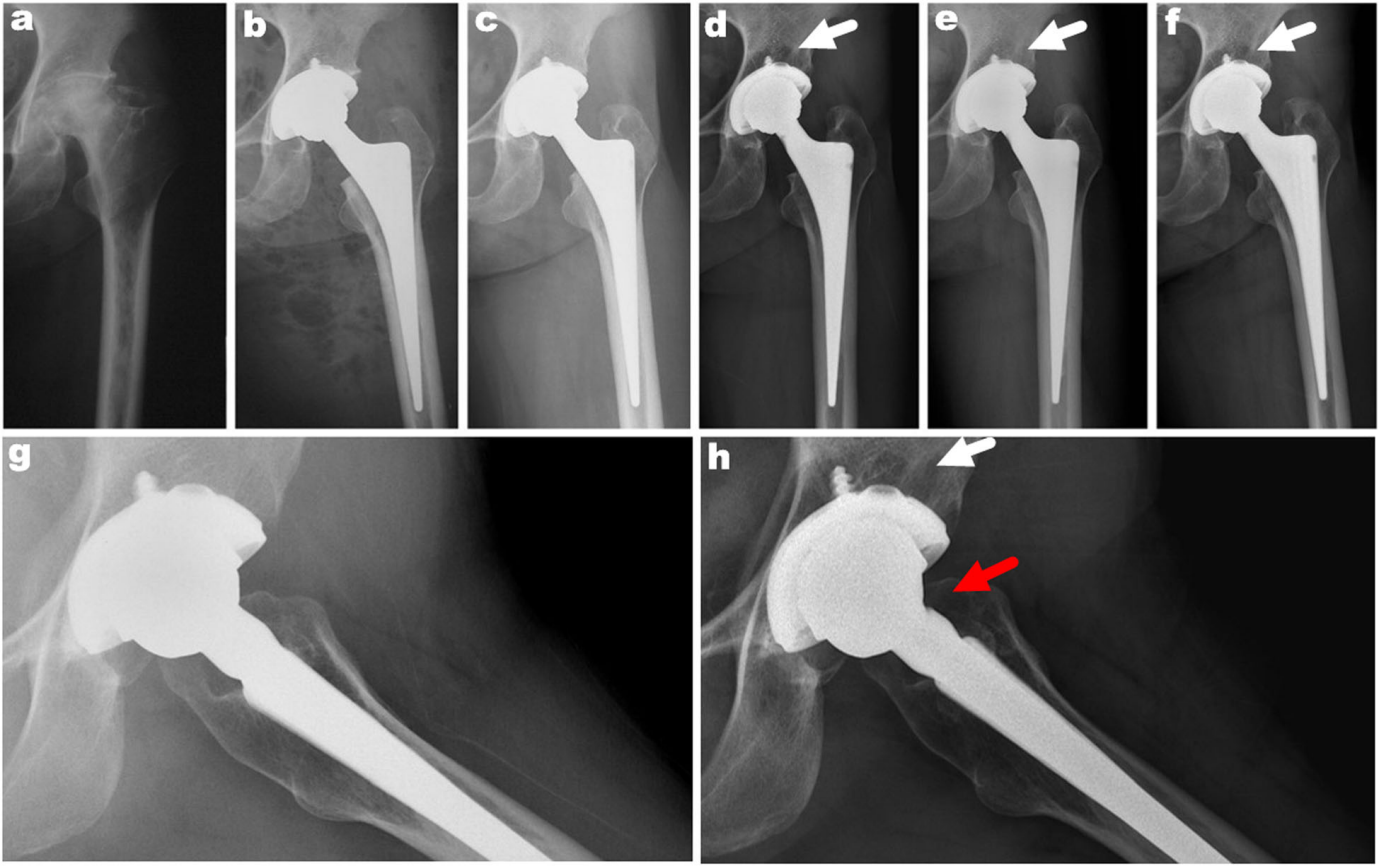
a Preoperative anteroposterior radiograph of the left hip showing avascular necrosis of the femoral head. b Immediate postoperative anteroposterior radiograph showing a well-fixated metal-on-metal articulated total hip arthroplasty. c–f An anteroposterior radiograph of the right hip at 3, 5, 11, and 22 years after operation, 5 years after surgery we can see osteolysis around the cup (white arrow). g Immediate postoperative frog-view radiograph. h A frog-view radiograph of the right hip at 22 years after operation, showing an obvious notch at the anterior part of the neck of the femoral stem (red arrow)
